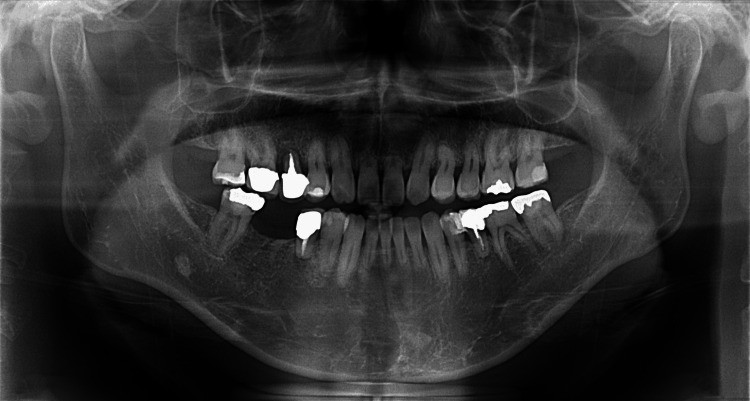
Panoramic radiograph after extraction of the distal root of the lower right first molar
